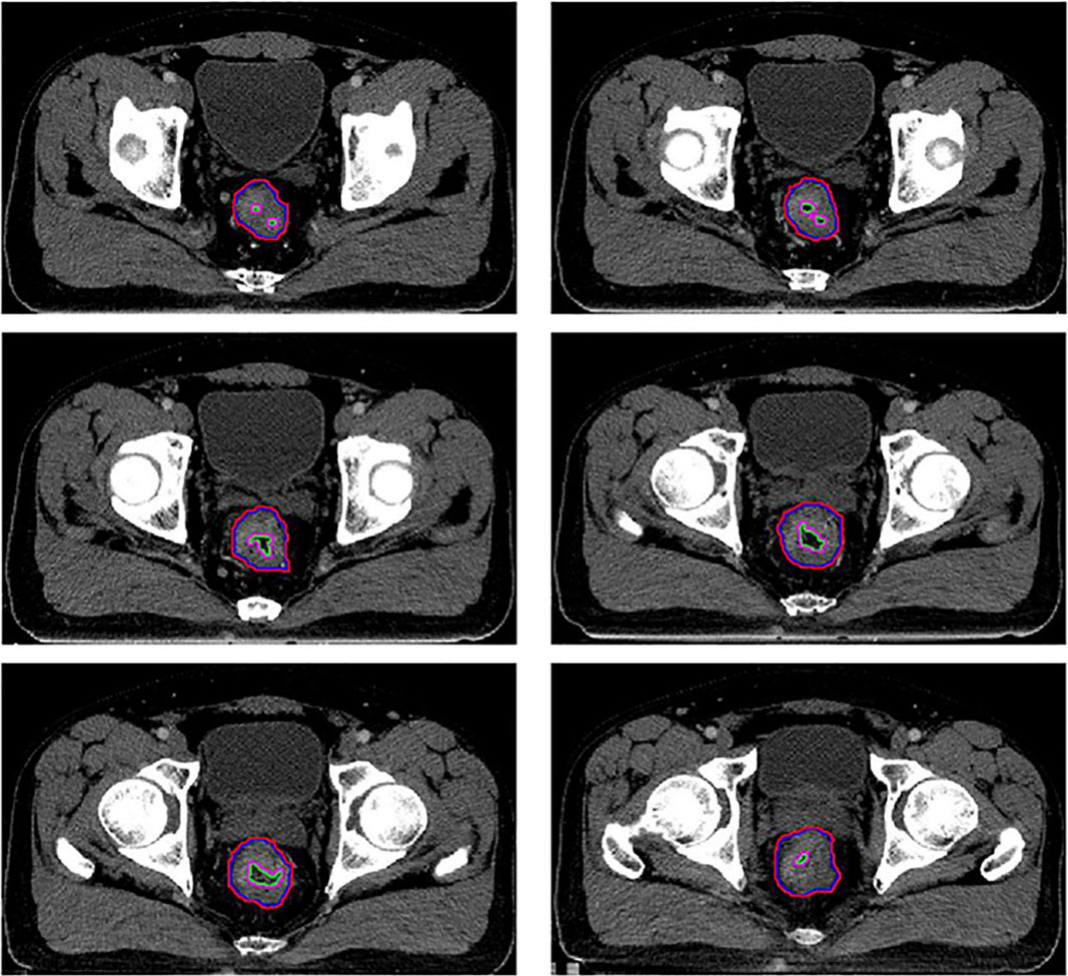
The rectal tumor of one representative patient on the axial images (contrast-enhanced CT). The red line encompasses the ATV area of every cross-sectional slice. The ATV–1 mm, the blue line, was generated from the ATV with a three-dimensional universal contraction of 1 mm length. The TSAO is the area between the red line and the blue line, encompassing the 1 mm layer volume. The green line encompasses the ITC area of every cross-sectional slice. The ITC+1 mm, the pink line, was generated from the ITC with a three-dimensional universal enlargement of 1 mm length. TSAI is the area between the pink line and the green line, encompassing the 1 mm layer volume. TSA is the sum value of TSAO and TSAI. The area between the red line and green line is the RTV. ATV, approximate tumor volume; TSAO, the tumor surface area outside the intestine; TSAI, the tumor surface area inside the intestine; TSA = TSAI + TSAO; RTV, real tumor volume.
